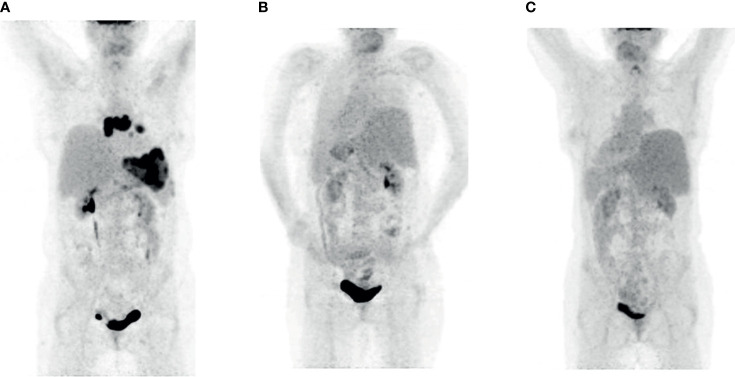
FDG PET imaging patient 2. (A) Relapse seven months after the therapy with CAR T-cells (prior to Pola-BR). (B) Complete metabolic remission after three courses of Pola-BR. (C) Confirmed complete metabolic remission 52 days after alloHSCT.
